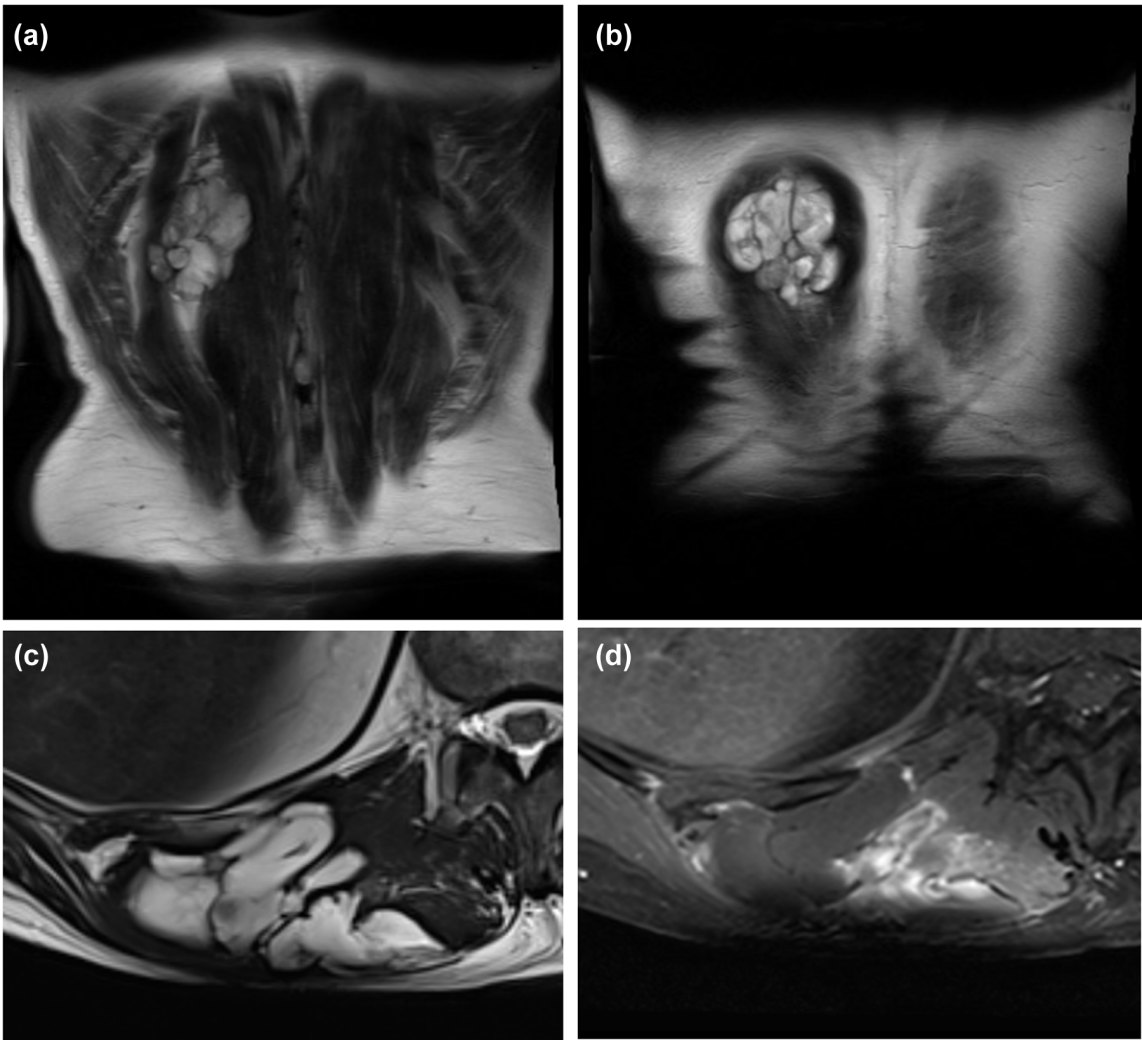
The MRI image of a contrast-enhanced coronal view of myxofibrosarcoma: (a, b) a soft tissue mass adhering to the skin and (c, d) a nodular cystic lesion depicting the size of the tumor with a large myxoid component. MRI-magnetic resonance imaging.
